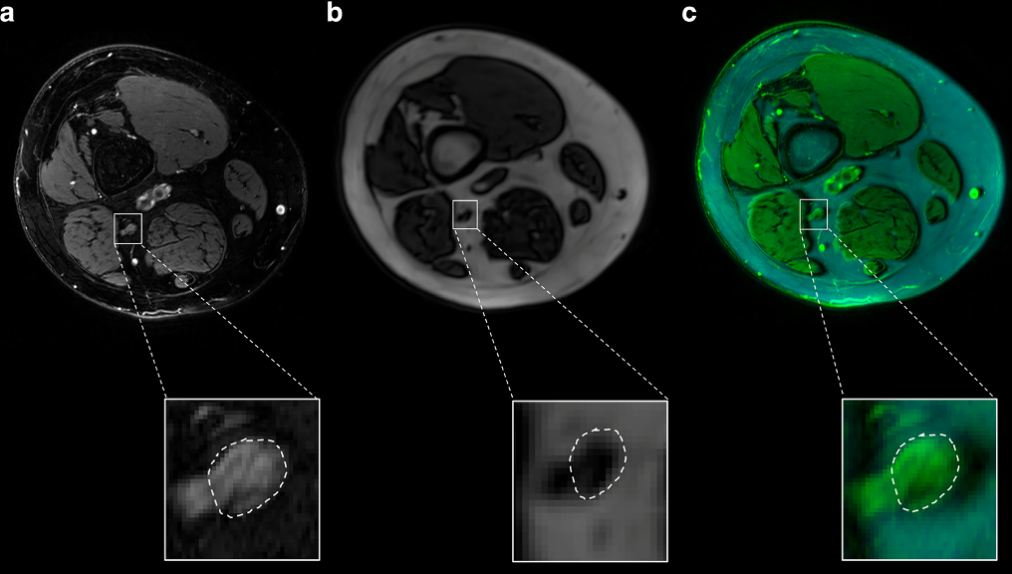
The process of coregistration of MRN sequences of a patient with T2D and small fiber neuropathy. An enlarged image of the sciatic nerve with encircled tibial compartment (*white dashed lines*) is shown highlighted by *white squares*. a Axial T2-weighted, fat-suppressed sequence at thigh level. No T2w-hyperintense or hypointense lesions, that would be a feature of large fiber neuropathy, can be seen in this T2D patient with SFN. b Axial T1-weighted VIBE sequence. c Coregistered image of T2-weighted (*green*) and VIBE (*cyan*) sequences
